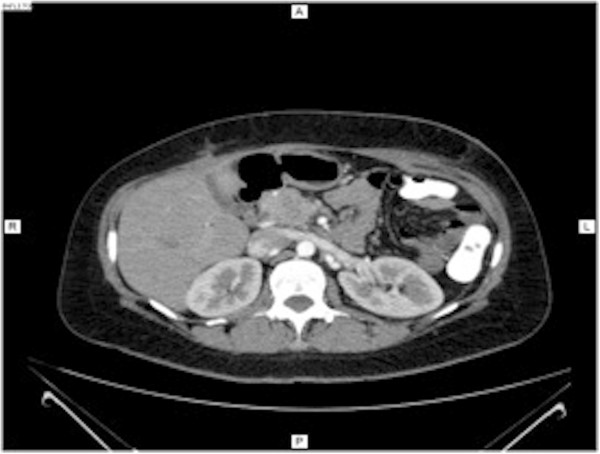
Computed tomography imaging after surgical resection of huge splenic hamartoma.
